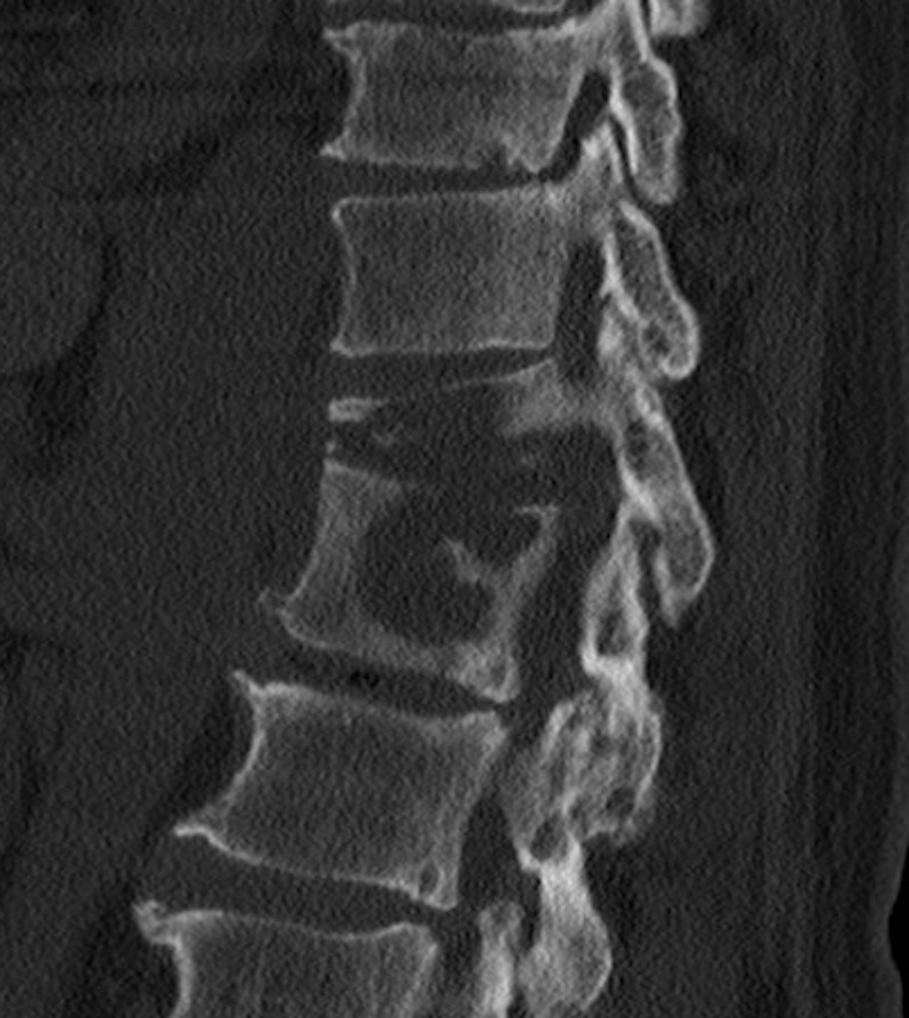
Preoperative sagittal CT Preoperative sagittal CT demonstrating erosive, lytic changes of the T11 and T12 vertebrae with compression deformity of the T11 vertebra.
